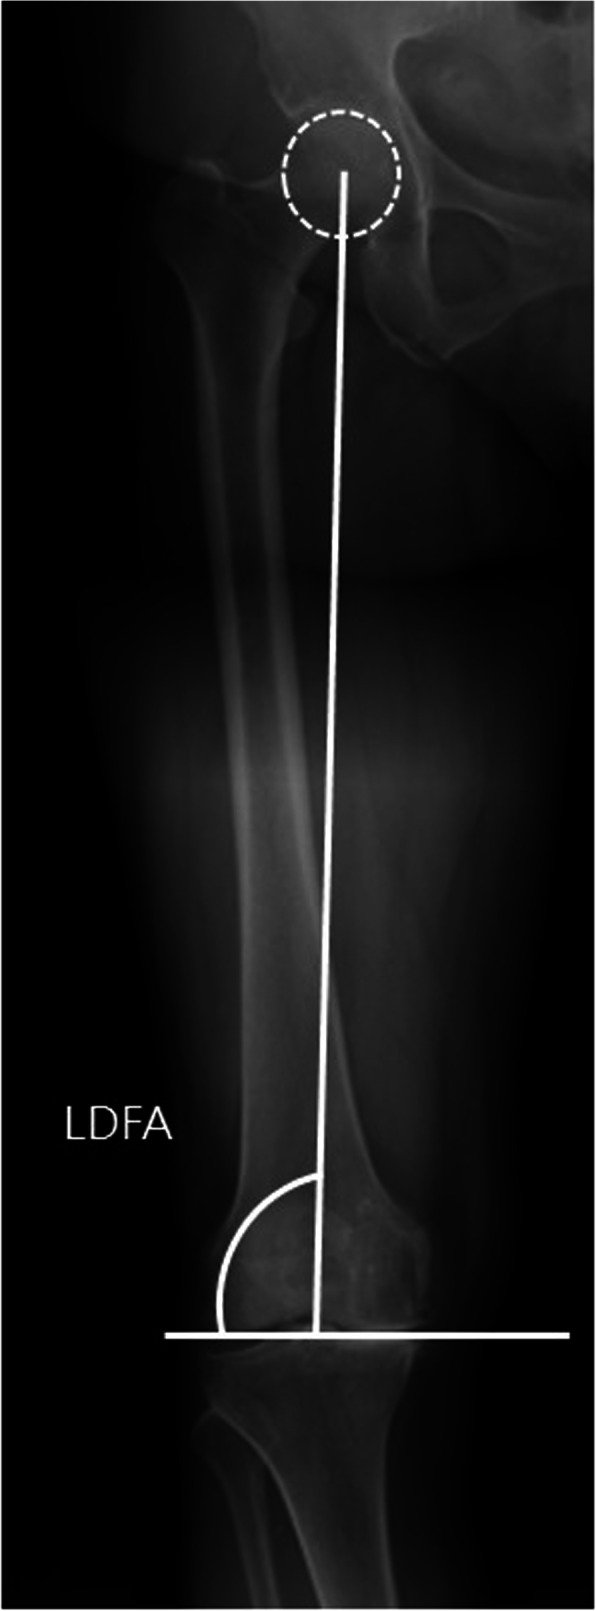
The lateral distal femur angle (LDFA) is measured on the whole-leg weight-bearing radiograph. It is defined as the lateral angle formed between the mechanical axis line of the femur (direct line from the center of femoral head to the top of the femoral notch) of the femur and knee joint line of the femur
